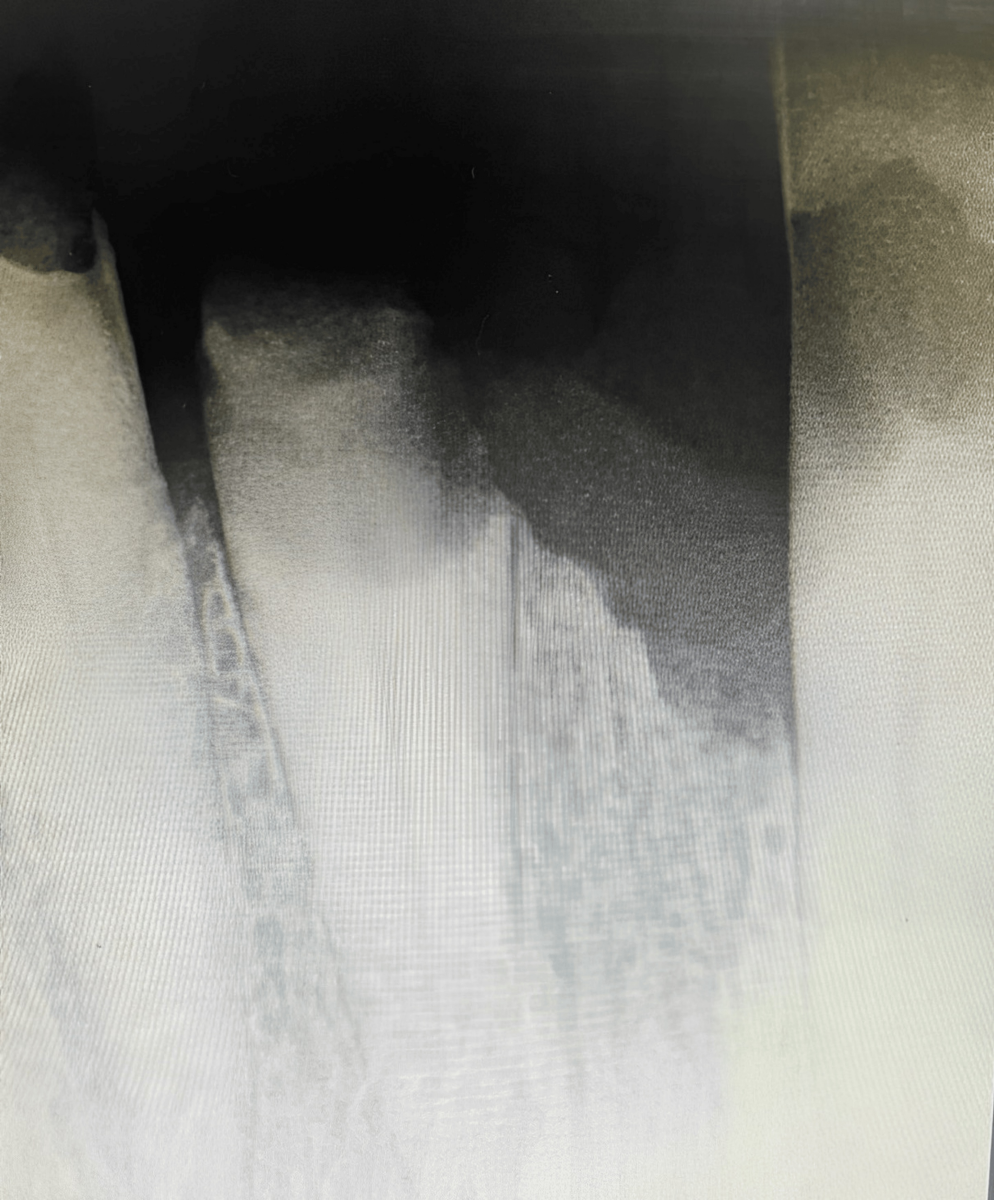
Radiographic view of the treated tooth taken on the seventh day.
